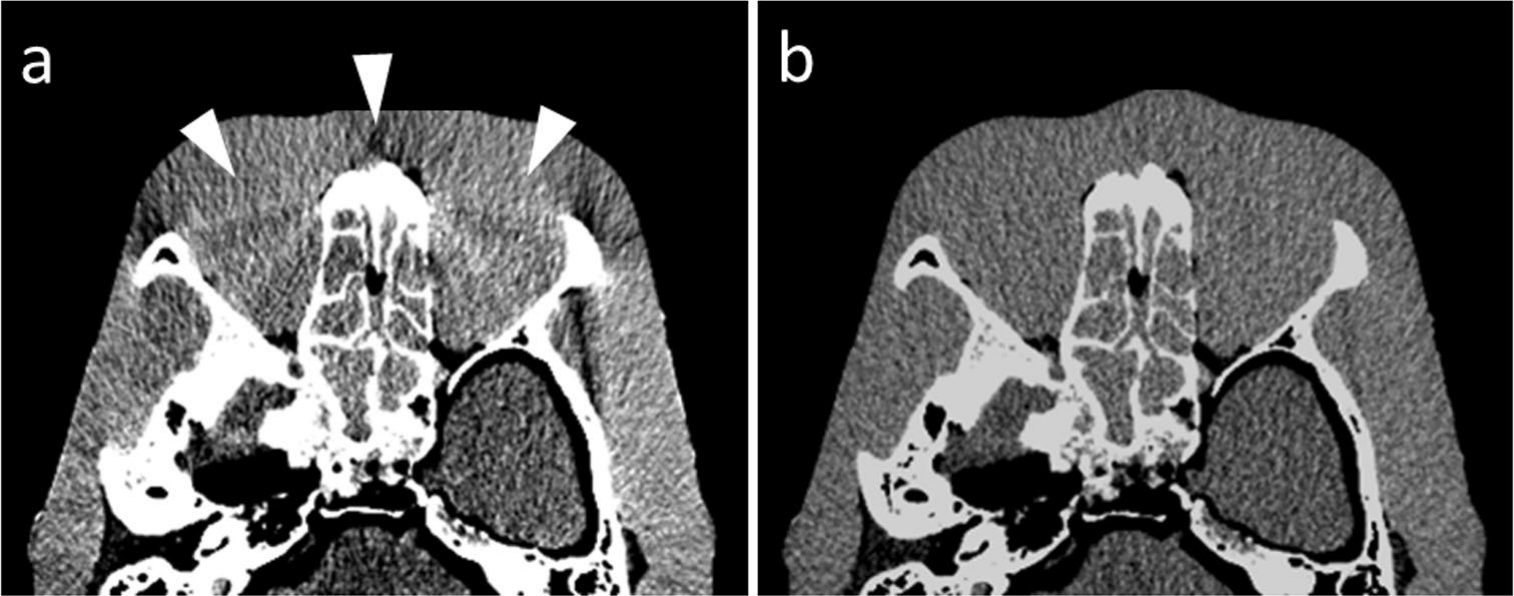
CT image processed with image-based analysis (a) and raw data-based analysis (b). In image-based analysis, beam-hardening artifacts from facial bones degrade the image quality (arrowheads). As the CT image processed with raw data-based analysis rather than image-based analysis exhibits lower beam-hardening artifacts, the acquired CT number would be accurate
